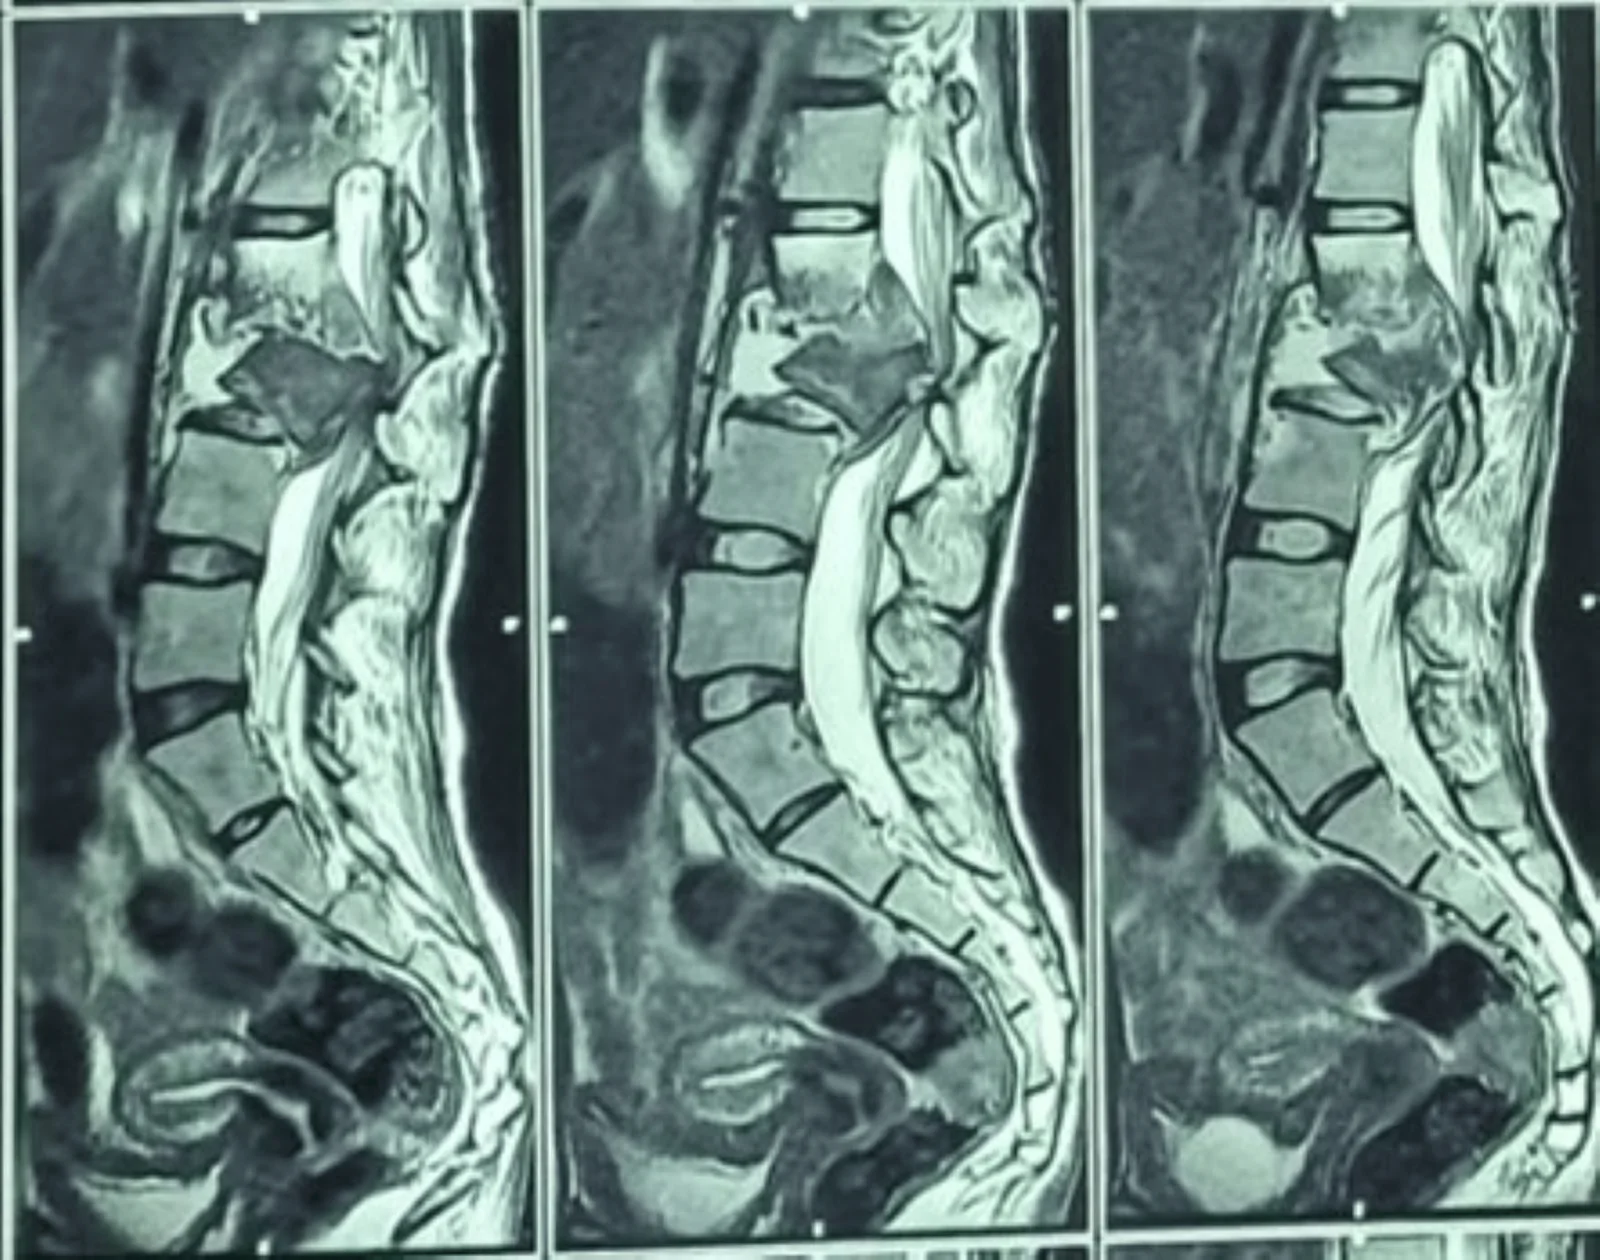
MRI of the thoracolumbar spine showing L3 vertebral collapse with retropulsion and posterior element involvement in a background of diffuse T2 hypointense marrow, favoring chronic healed tuberculous spondylitis with resultant instability and reduced spinal canal space.
